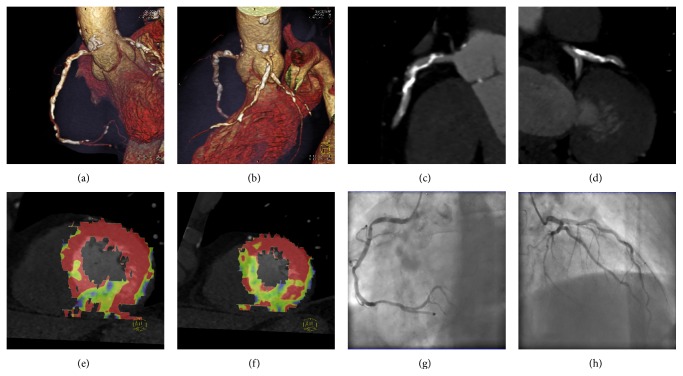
(a–h) 61-year-old male with severe occlusions of iliac arteries was referred to aortobifemoral bypass and without history of CHD symptoms underwent complete CT protocol: coronary CT angiography (CTA), stress CT perfusion (CTP), and rest CTP. Heart rate (HR) during CTA was 71/min (3.1 mSv) and 96/min during stress CTP (5.3 mSv) after 400 micrograms of regadenoson application (60 sec interval). Rest CTP (7.9 mSv) was performed 15 min after stress CTP and HR was 75/min. ICA was performed with 1-day interval. Volume rendering technique images (a, b) show calcified plaques in proximal parts of coronary arteries. Multiplanar reformation images (c, d) show irregular stenosis of the right coronary artery (RCA) and left anterior descending (LAD) artery described as significant using CTA. Colour-coded maps (e, f) of stress myocardial perfusion (blood volume) show perfusion defect in RCA territory and normal perfusion in LAD territory (complete perfusion recovery in rest myocardial perfusion). Invasive coronarography confirmed significant stenosis of RCA (g) and just mild irregularity on LAD (h) artery, CTA decision correctly reclassified by CTP.
